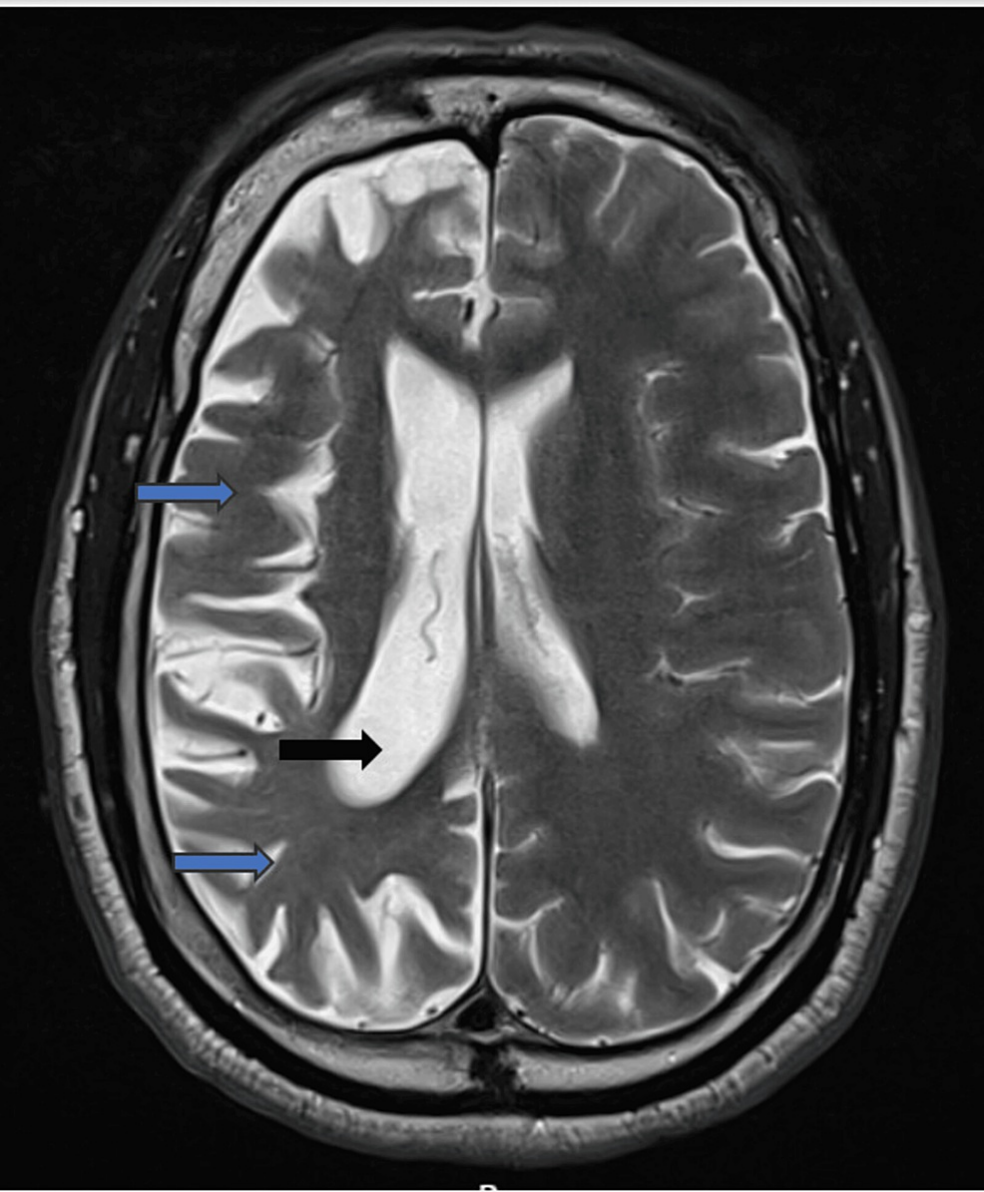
T2/fluid-attenuated inversion recovery MRI of the head axial section revealing right cerebral cortical atrophy (blue arrows) and a comparatively enlarged right lateral ventricle (black arrow).
